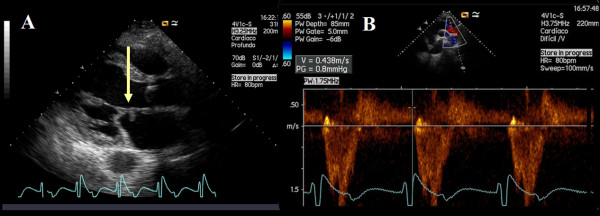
Transthoracic echocardiogram - parasternal long-axis view with evidence of a nodular image attached to the atrial side of the anterior leaflet of the mitral valve (A, arrow) and a holodiastolic flow reversal in the descending thoracic aorta recorded from a suprasternal notch window with terminal velocity > 20 cm/s (B) supporting severe aortic regurgitation.
